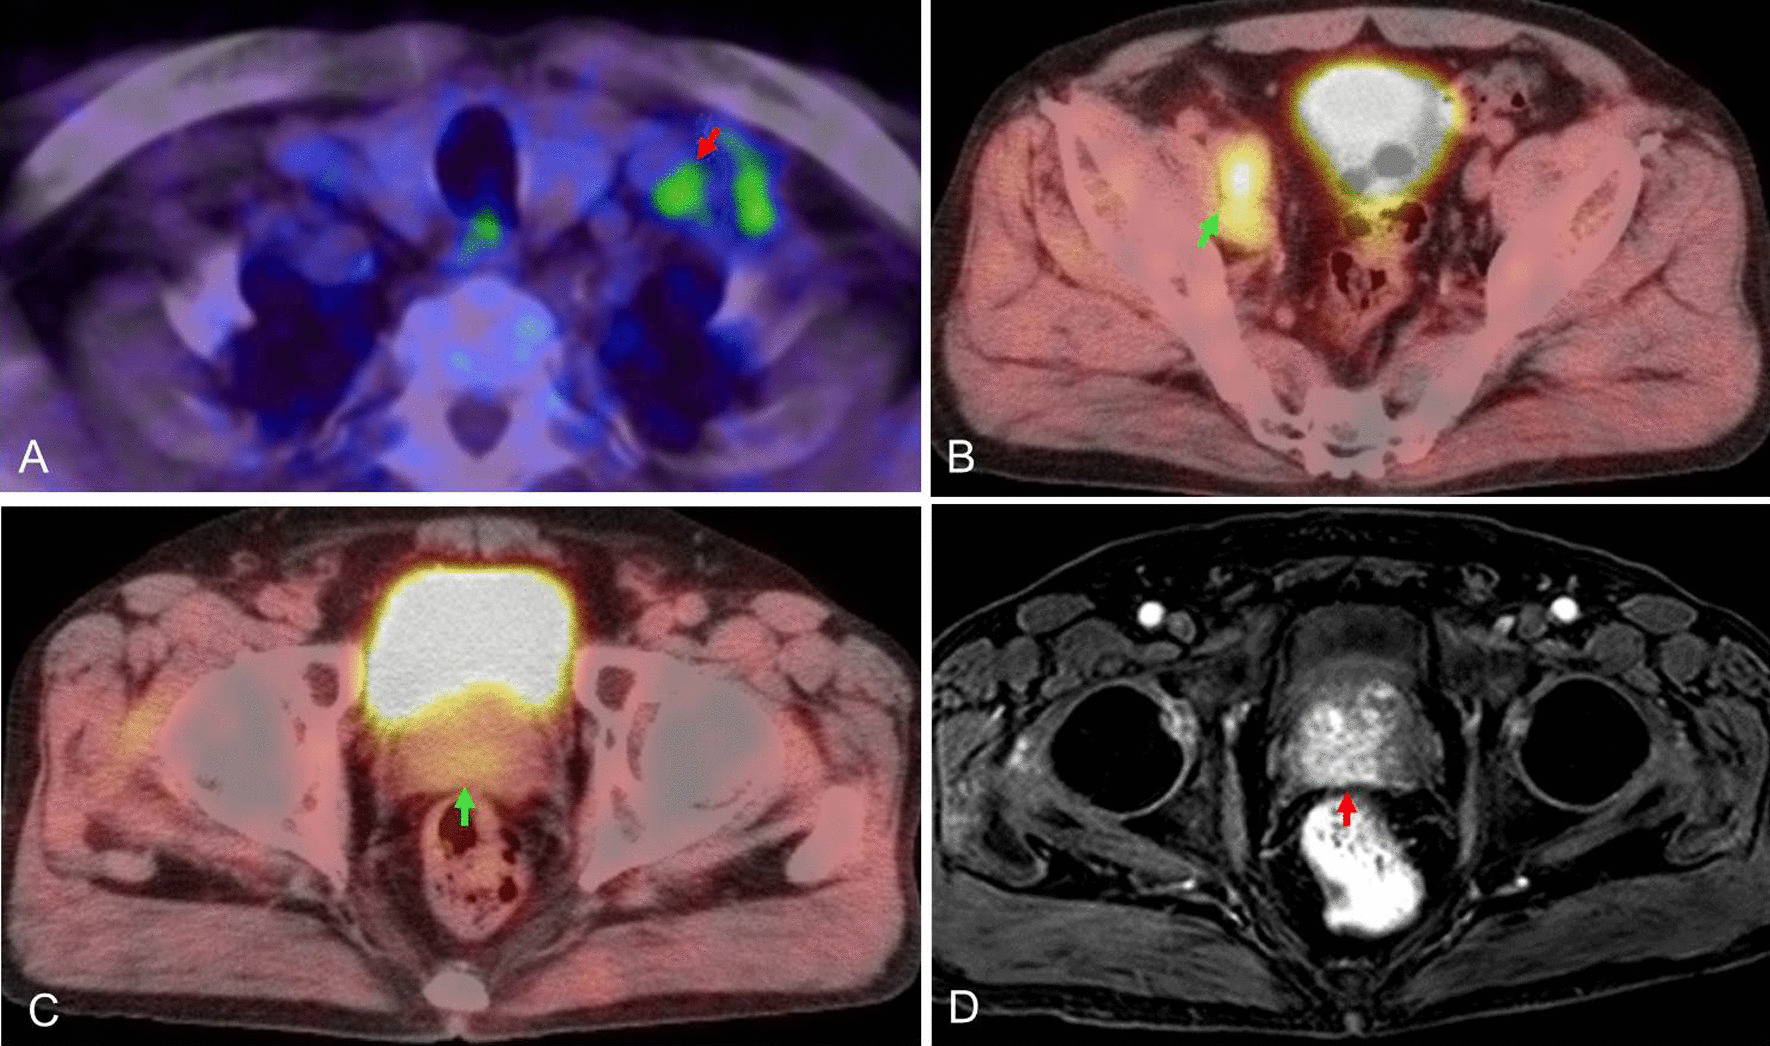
^18^F-FDG PET/CT and MRI findings. A
